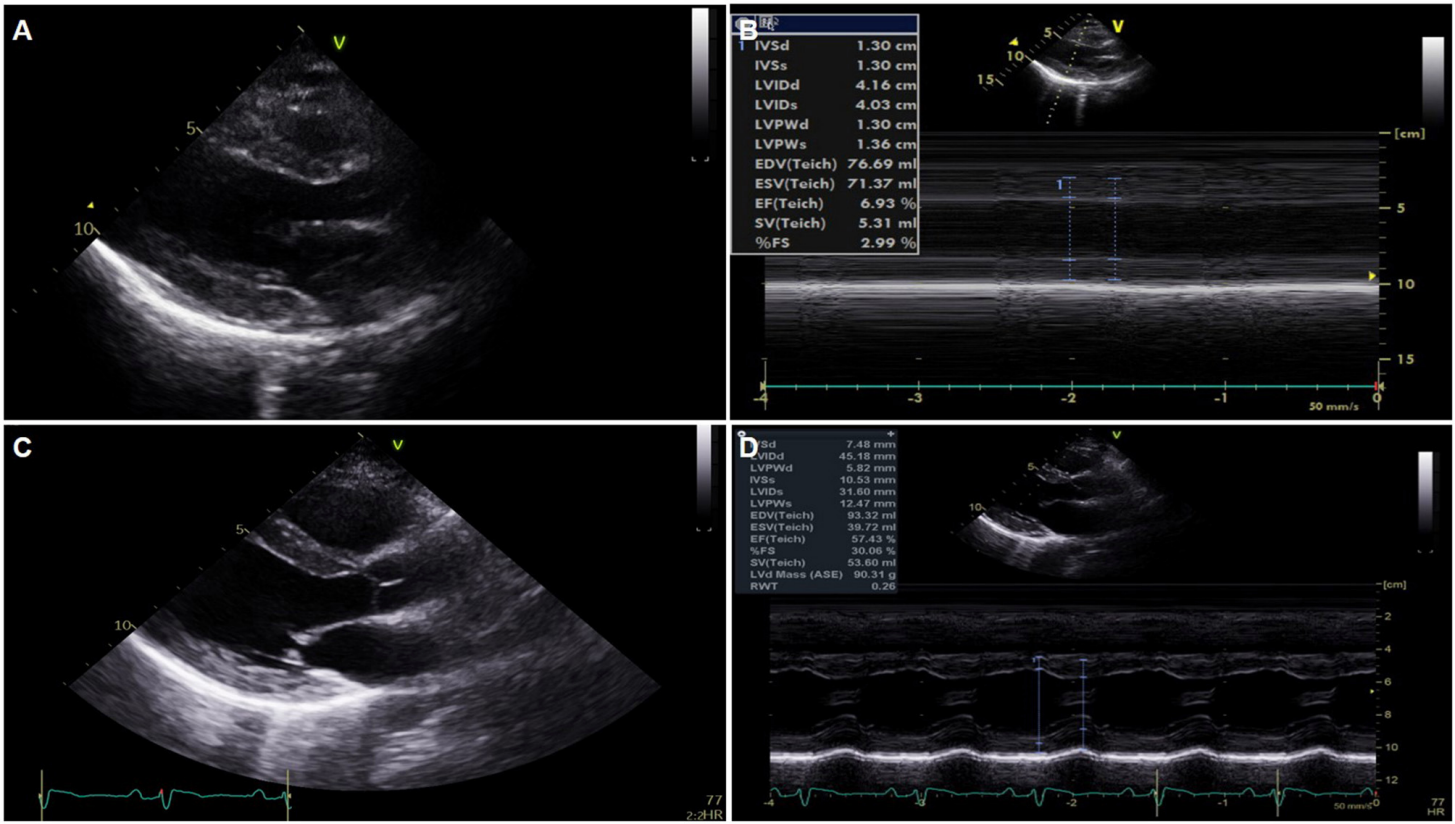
Serial changes of echocardiography. (A,B) Global akinesia with severe left ventricular (LV) dysfunction and marked edematous left ventricular wall thickening on portable echocardiography performed just after extracorporeal membrane oxygenation application. (C,D) Normalized LV function and wall thickness on pre-discharge echocardiography.
